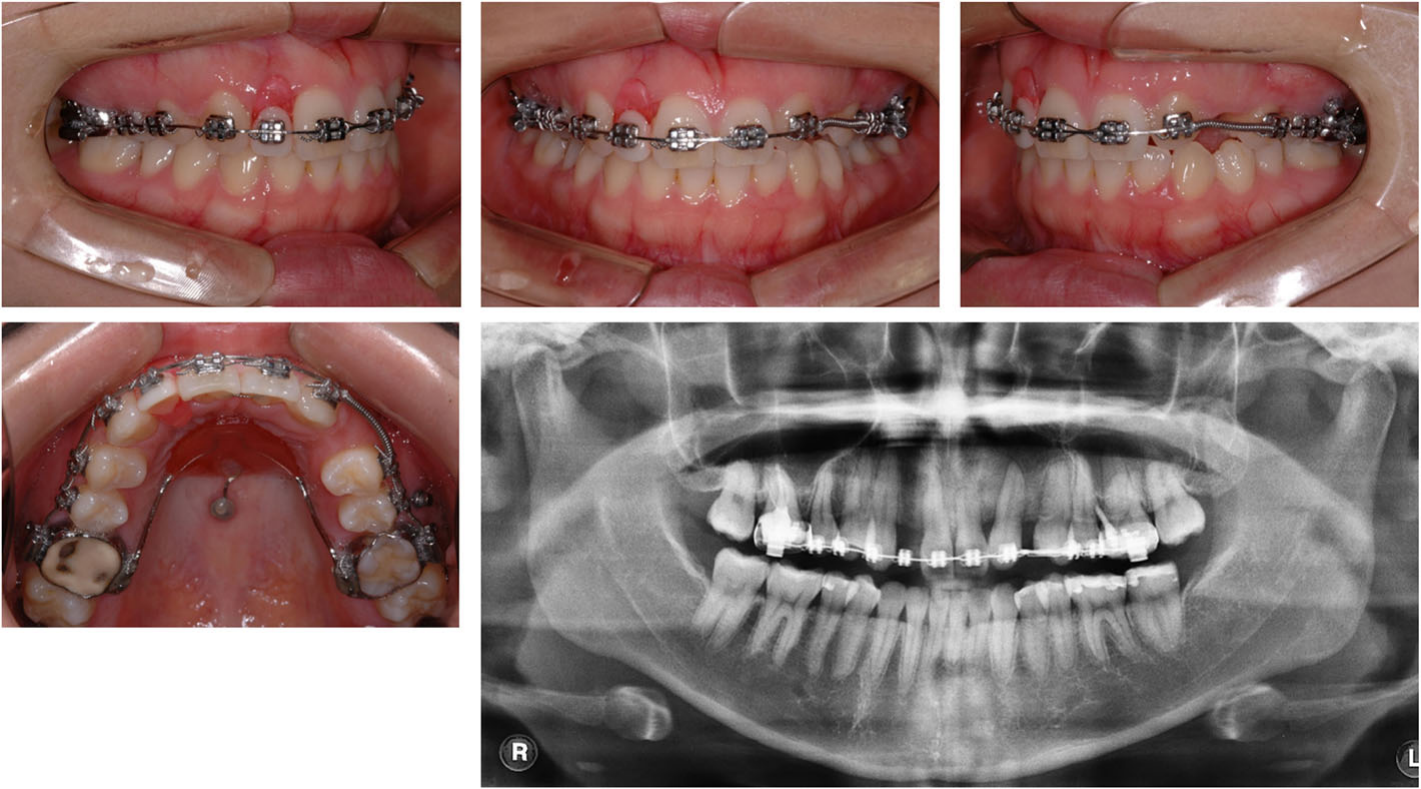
Intraoral photographs and panoramic radiographs after 9 months of space opening treatment
